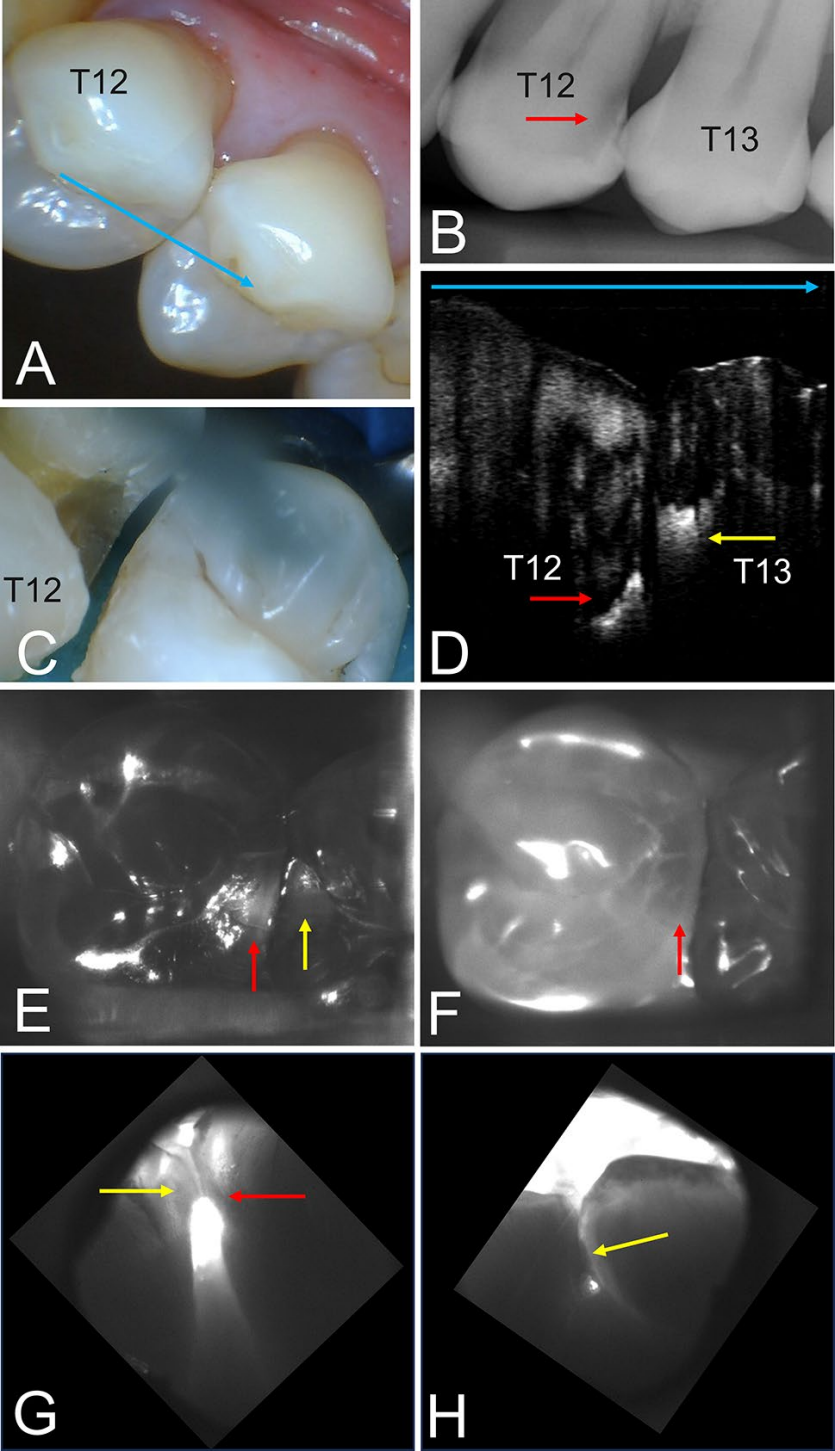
(A) Color image of the contact between tooth #12 (T12) and tooth #13 (T13), no lesions are visible. (B) Radiograph shows a large proximal lesion (red arrow) on tooth#12 at the distal contact that was scheduled for restoration. No lesion is visible on the opposing mesial contact of tooth #13. (C) After the cavity preparation decalcification is now visible on the mesial contact of tooth#13. (D) A CP-OCT b-scan image taken from the occlusal surface above the contact along the path of the blue arrow shown in (A) shows both proximal lesions that appear with increased subsurface reflectivity at the positions indicated by the red and yellow arrows. The CP-OCT scan shows the intensity with depth and lateral position with white high intensity and black low intensity. (E) SWIR-R occlusal reflectance shows lesions on both surfaces (red and yellow arrows) while (F) SWIR-OT only shows the lesion that was restored. (G) SWIR-PT image taken from the buccal side shows both proximal lesions across the contact while the (H) SWIR-PT image taken from the lingual shows only the lesion on tooth #13
